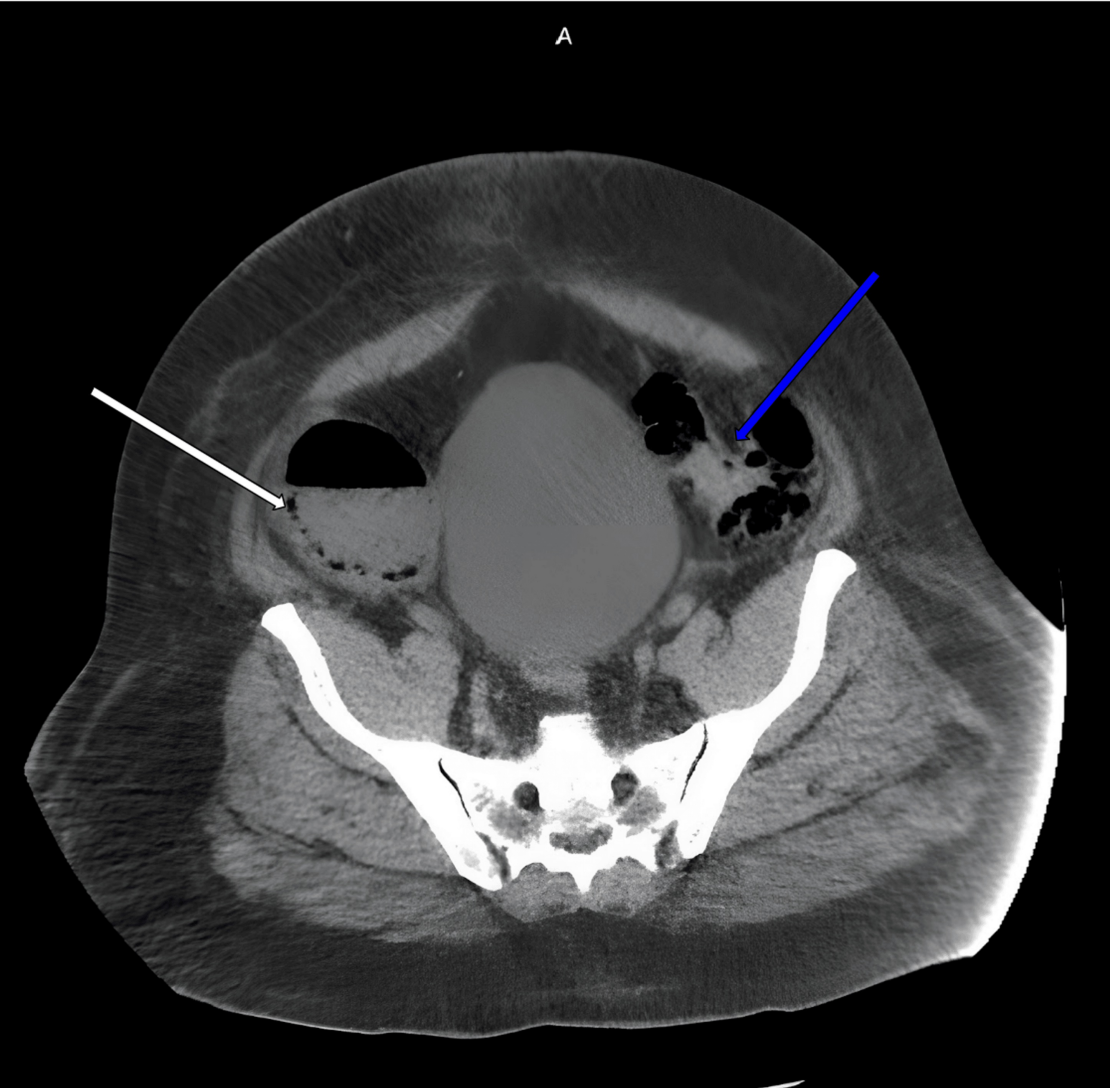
Transverse CT scan before colectomy showing pneumatosis at the ascending colon (white arrow) and the obstructing mass at the sigmoid colon (blue arrow) CT: computed tomography
